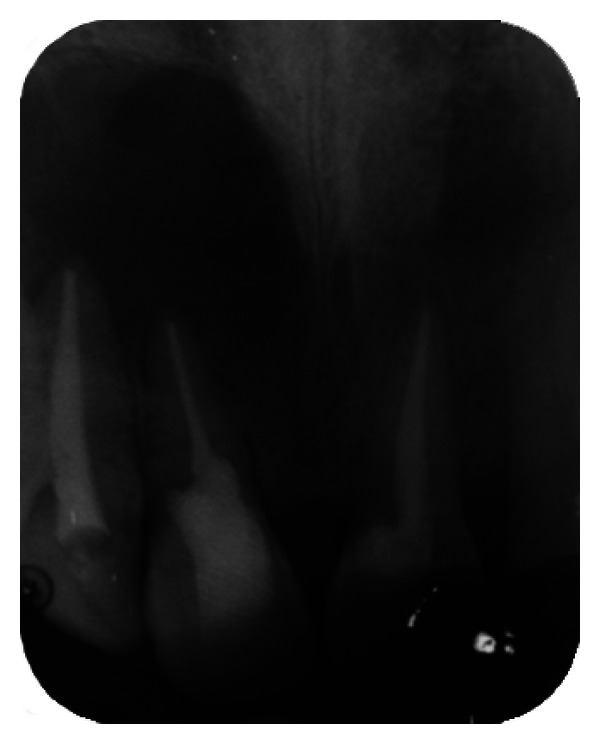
Postobturation IOPA radiograph.
